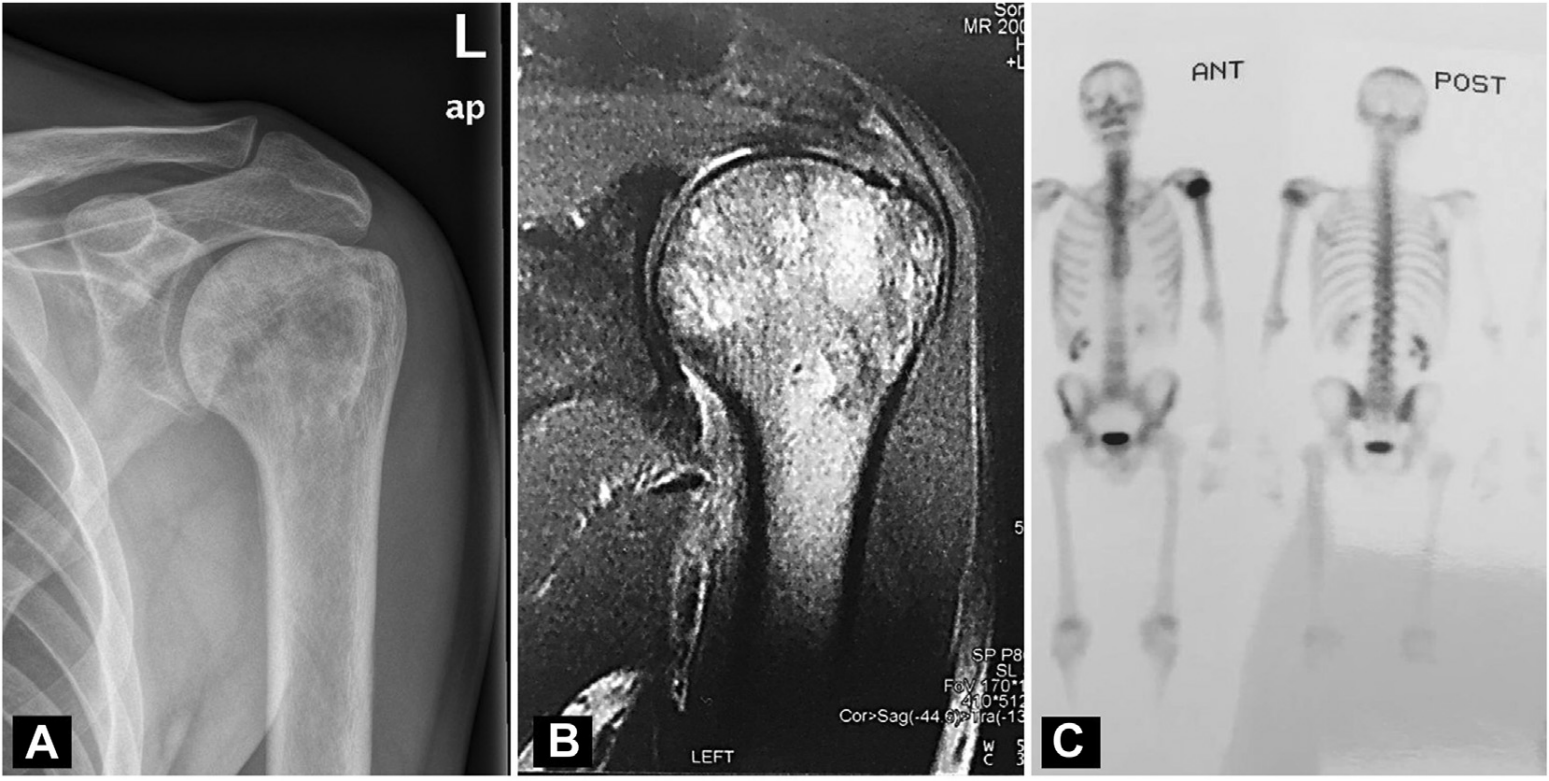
(A) Plain X-ray of the left shoulder showing a permeative blastic-sclerotic pattern within the humeral head without cortical thickening and/or periosteal reaction, (B) MRI of the shoulder showing the same blastic-sclerotic pattern and some narrowing of the subacromial space, raising the possibility of rotator cuff impingement, (C) 3-phase bone scan showing significant uptake in the left proximal humerus. *MRI*, magnetic resonance imaging.
